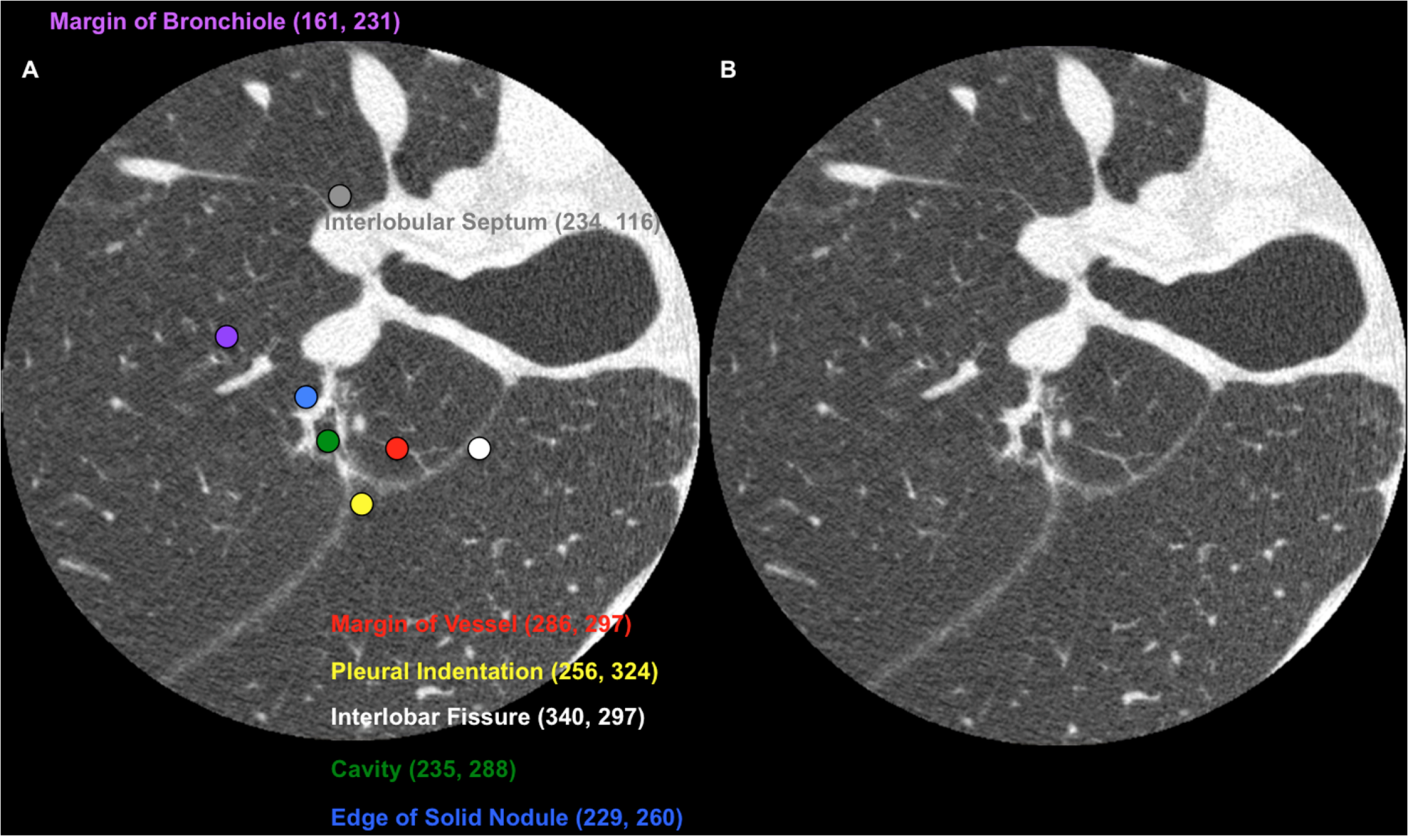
Example of CT images for the observer test. (A) An example of an ultra-high-resolution CT image with different colored dots and annotations used for the observer test. The X and Y coordinates of each dot are shown in parentheses. The image shows a lepidic-predominant invasive adenocarcinoma with a tumor size of 1.2 x 0.9 x 0.9 cm located in segment 2 of the right upper lobe in a 73-year-old man. The blue dot indicates the edge of a solid nodule. The green dot indicates a cavity. The yellow dot indicates pleural indentation of the interlobar fissure. The white dot indicates a fissure between the right upper lobe and the right lower lobe. The red dot indicates the margin of the pulmonary vessel. The purple dot indicates the edge of a bronchiole. The gray dot indicates an interlobular septum. (B) Ultra-high-resolution CT image in which the dots and annotations are hidden.
